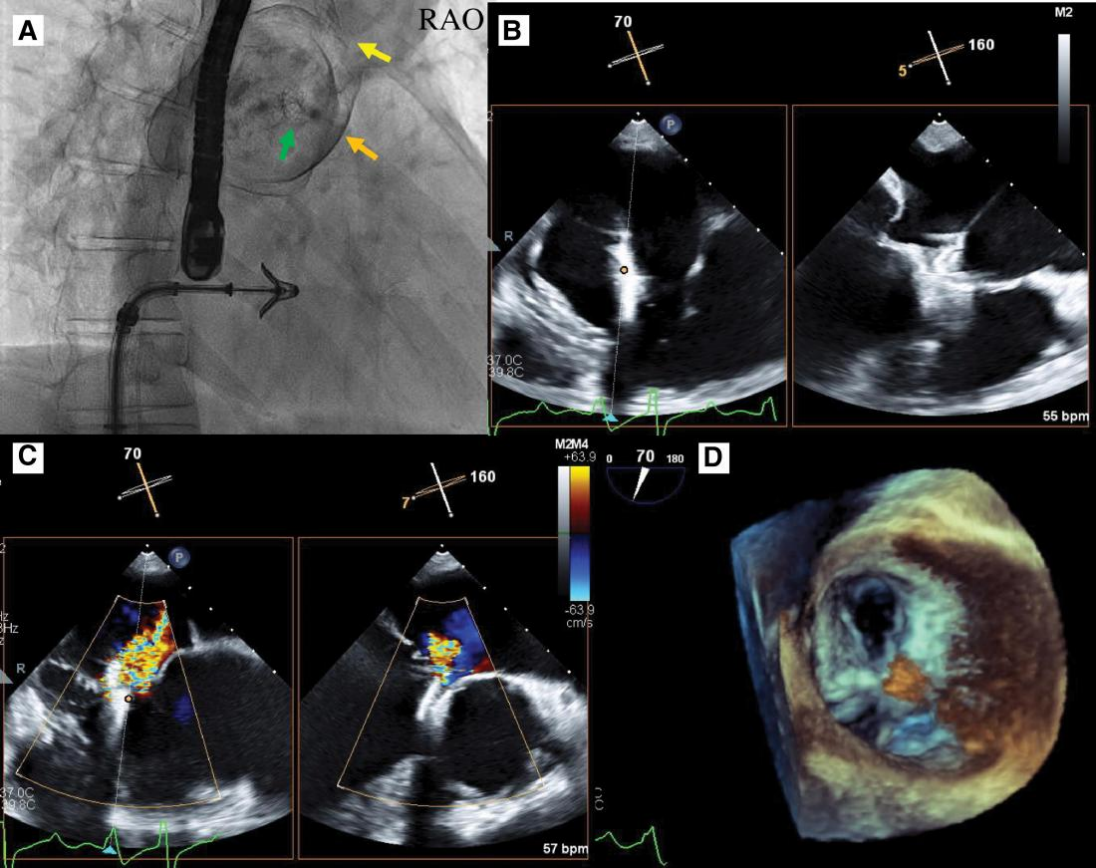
Procedural transesophageal echocardiography and fluoroscopic images. (*A*) Fluoroscopic image shows the MitraClip in a straddling position and stent implantation of an arterioportal shunt (green arrow) and patent ductus arteriosus (yellow arrow) with seroma formation (orange arrow). (*B*) Transesophageal echocardiography demonstrating an attempt to grasp two leaflets with the clip. (*C*) Post-procedural moderate tricuspid regurgitation. (*D*) Double-orifice tricuspid valve after MitraClip insertion. TEE, transesophageal echocardiography; RAO, right anterior oblique.
